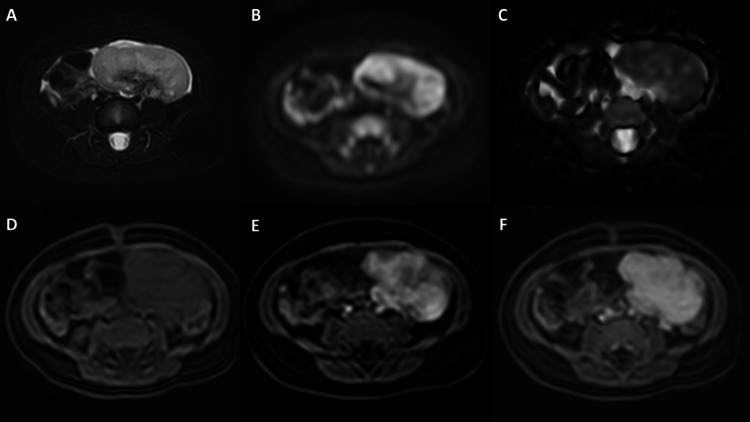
Abdominal and pelvic contrast MRI. Tumor originating in the left ovary, hyperintense on T2-weighted images (A), with a marked restriction on diffusion-weighted images (B) and the respective apparent diffusion coefficient (ADC) map (C). The tumor is isointense on T1-weighted images before gadolinium injection (D) and had intense and progressive enhancement in arterial (E) and venous (F) phases.
